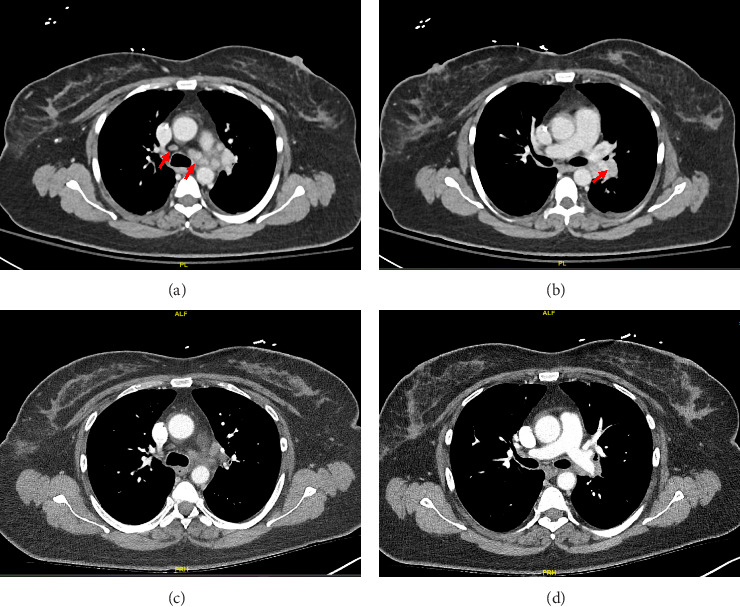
Contrast-enhanced chest computed tomography (CT), contrast-enhanced chest CT scan (a, b) showing enlarged lymph nodes in the mediastinum (a) and left hilum (b) after 8 cycles of neoadjuvant treatment. Contrast-enhanced chest CT scan (c, d) showing improved lymphadenopathy after corticosteroids treatment and 2 months after stopping immunotherapy in the mediastinum (c) and left hilum (d).
